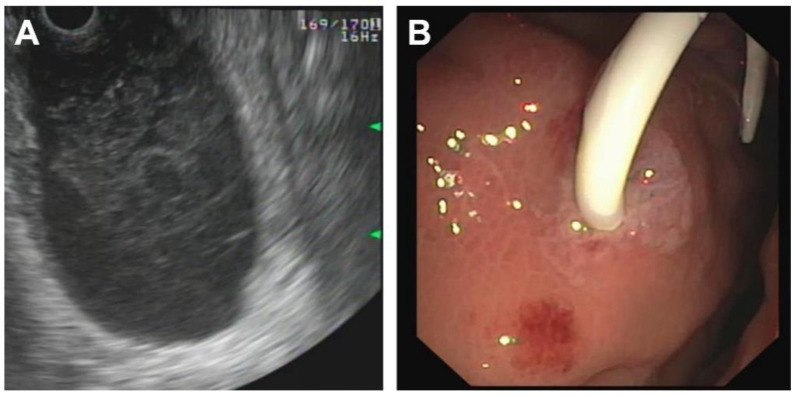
Transgastric endosonography showing a retention at the site of rupture (A). Transgastric placement of a pigtail drain (B).
